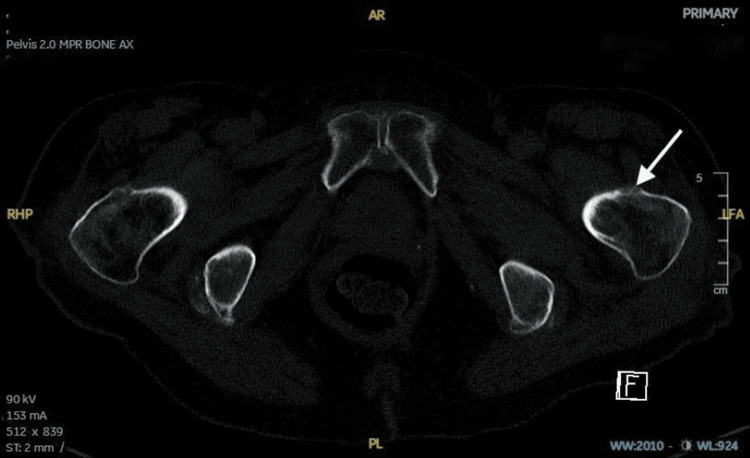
CT scan of the pelvis without contrast. Axial image: suspected hazy cortical outline with suspected cortical interruption along the anterior aspect of the left femoral neck CT: computed tomography
